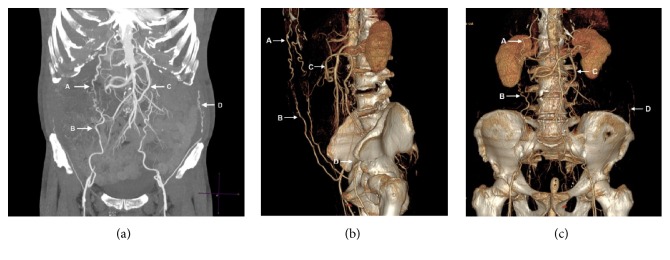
CT angiography and 3D reconstruction showing Winslow's pathway (A and B), the arc of Riolan (C), and the lower intercostal artery to circumflex iliac artery collateral route (D).
